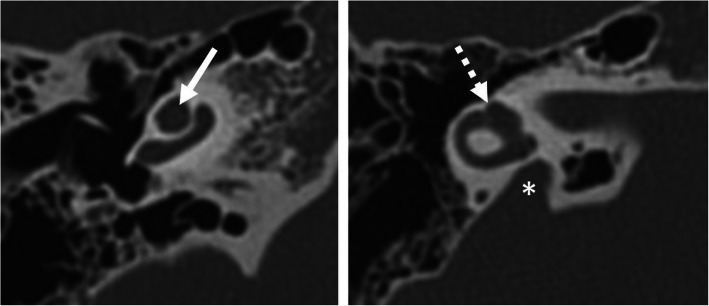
A 12-year-old male patient, with bilateral severe SNLH from birth. HRCT axial images show incomplete partition type II with the cystic apex of the cochlea (arrow) and enlarged vestibular aqueduct (asterisk). Vestibulum is minimally enlarged (dashed arrow), and semicircular canals appear normal
